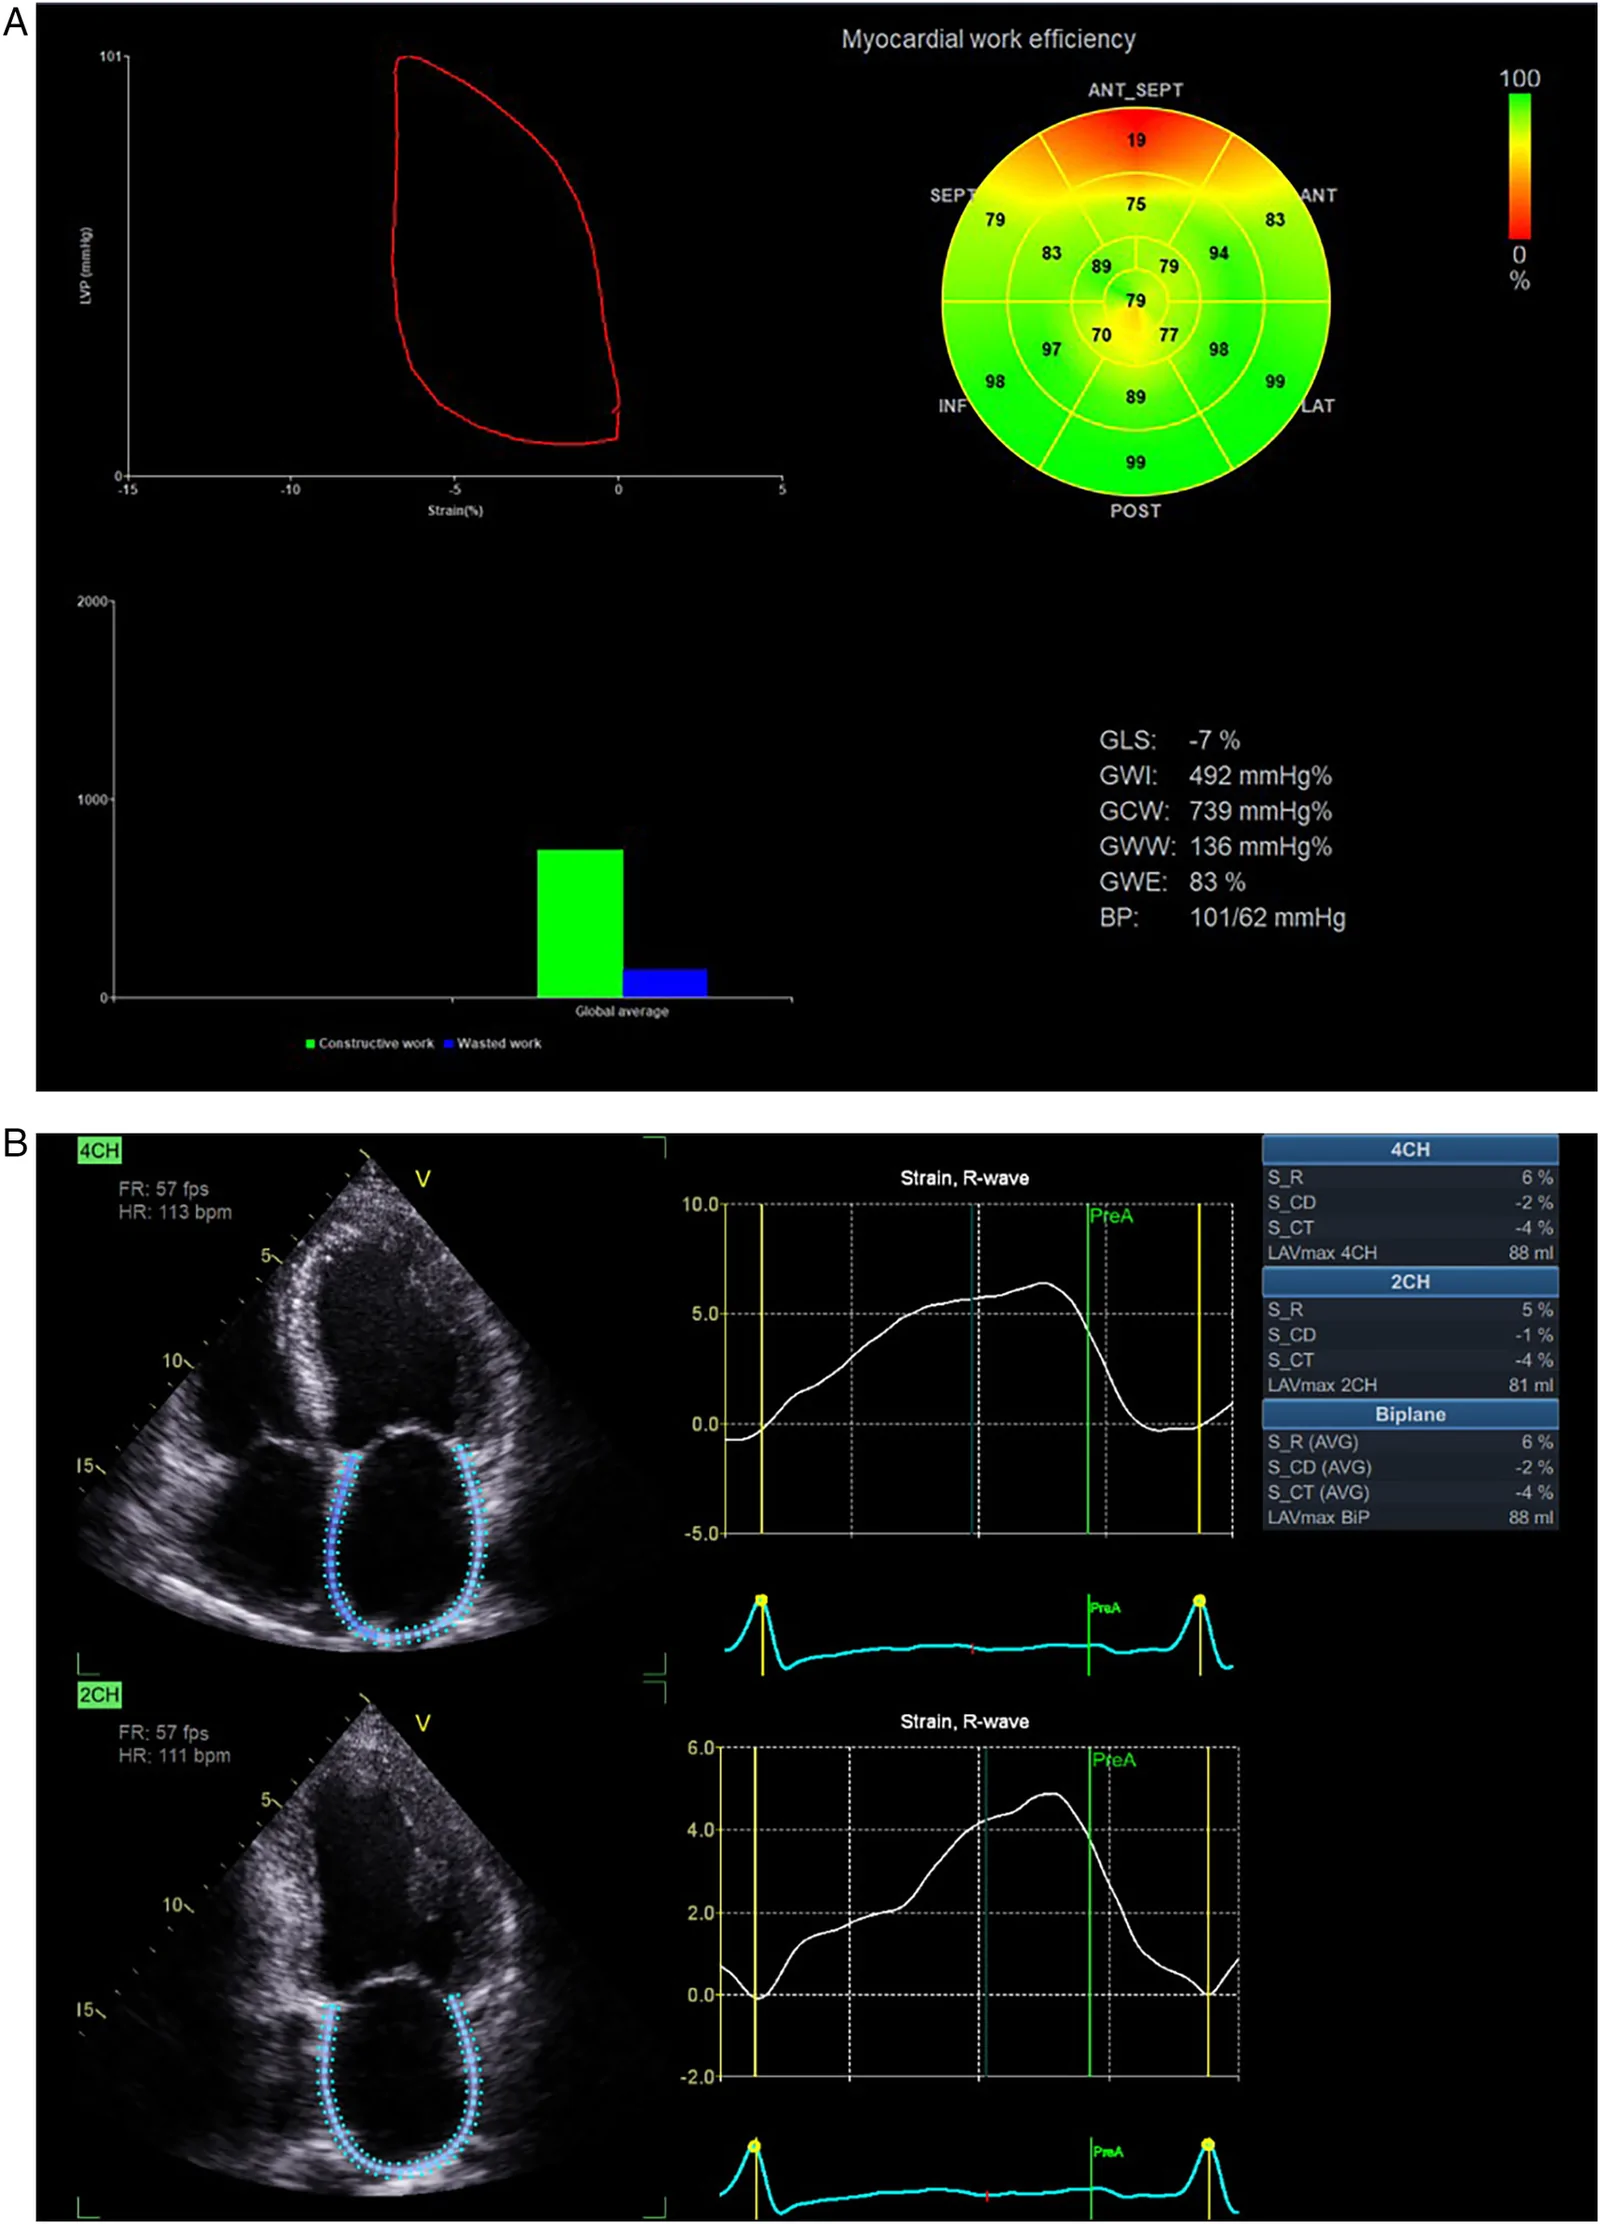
Representative speckle-tracking echocardiography images for GWE and LASr. (A) Bull's eye plot of global work efficiency (GWE) showing segmental distribution of myocardial work efficiency. (B) Left atrial reservoir strain (LASr) analysis from the apical four-chamber and two-chamber views. The strain curve depicts phasic changes in left atrial deformation; the peak during ventricular systole (LASr) reflects left atrial reservoir function.
